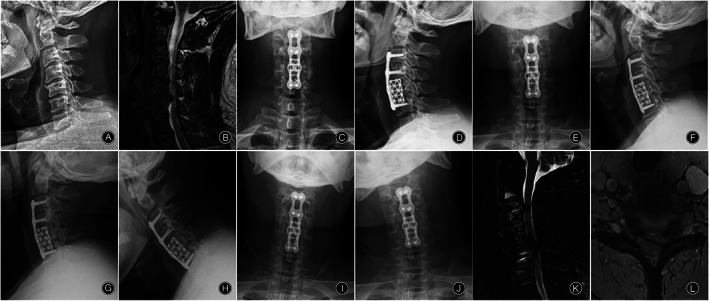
A 48‐year‐old male who underwent corpectomy ACDF hybrid procedures with a TMC for MDCM. Preoperative radiographs (A, B). 1 week X‐rays (C, D), 54 months X‐rays (E‐J) and 54 months MRI (K, L) postoperative follow‐up radiographs. 54 months after surgery, X‐rays (F‐H) showed osteophytes and narrowing of the intervertebral spaces at the adjacent segment (C6/7). Sagittal and axial MRI (K, L) at 54 months after surgery showed with disc herniation pressing on the spinal cord and nerves at the adjacent segment (C6/7).
